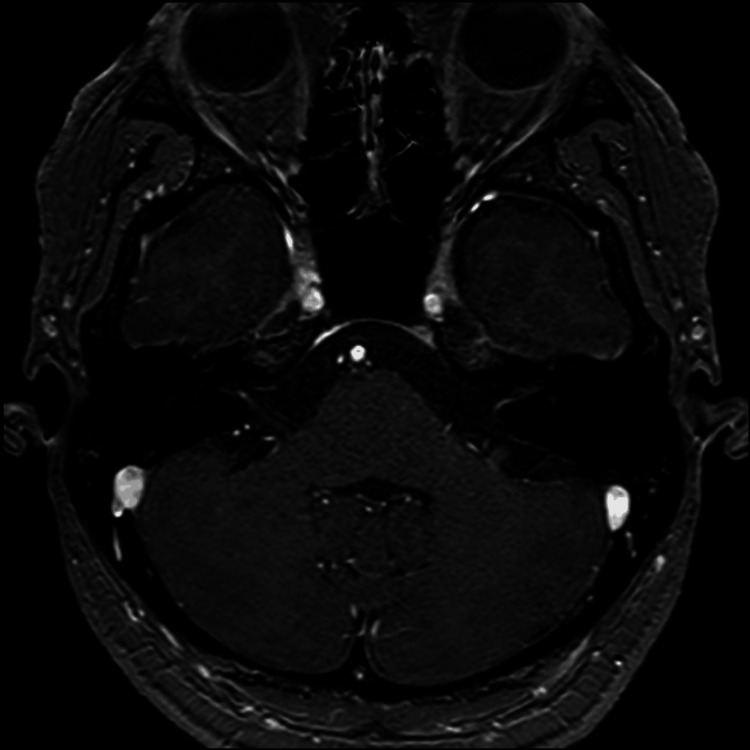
Contrast-enhanced MRI No obvious abnormal signals or contrast effects were observed in the internal auditory canal.
